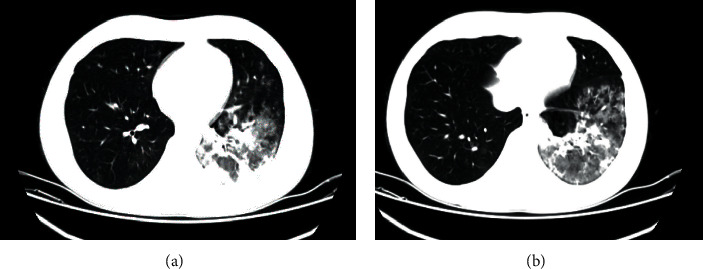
The patient's thorax CT images show radiologic findings consistent with COVID-19 pneumonia. Diffuse ground glass-like consolidated nodular lesions in the left hemithorax continue to the lower lobes.
